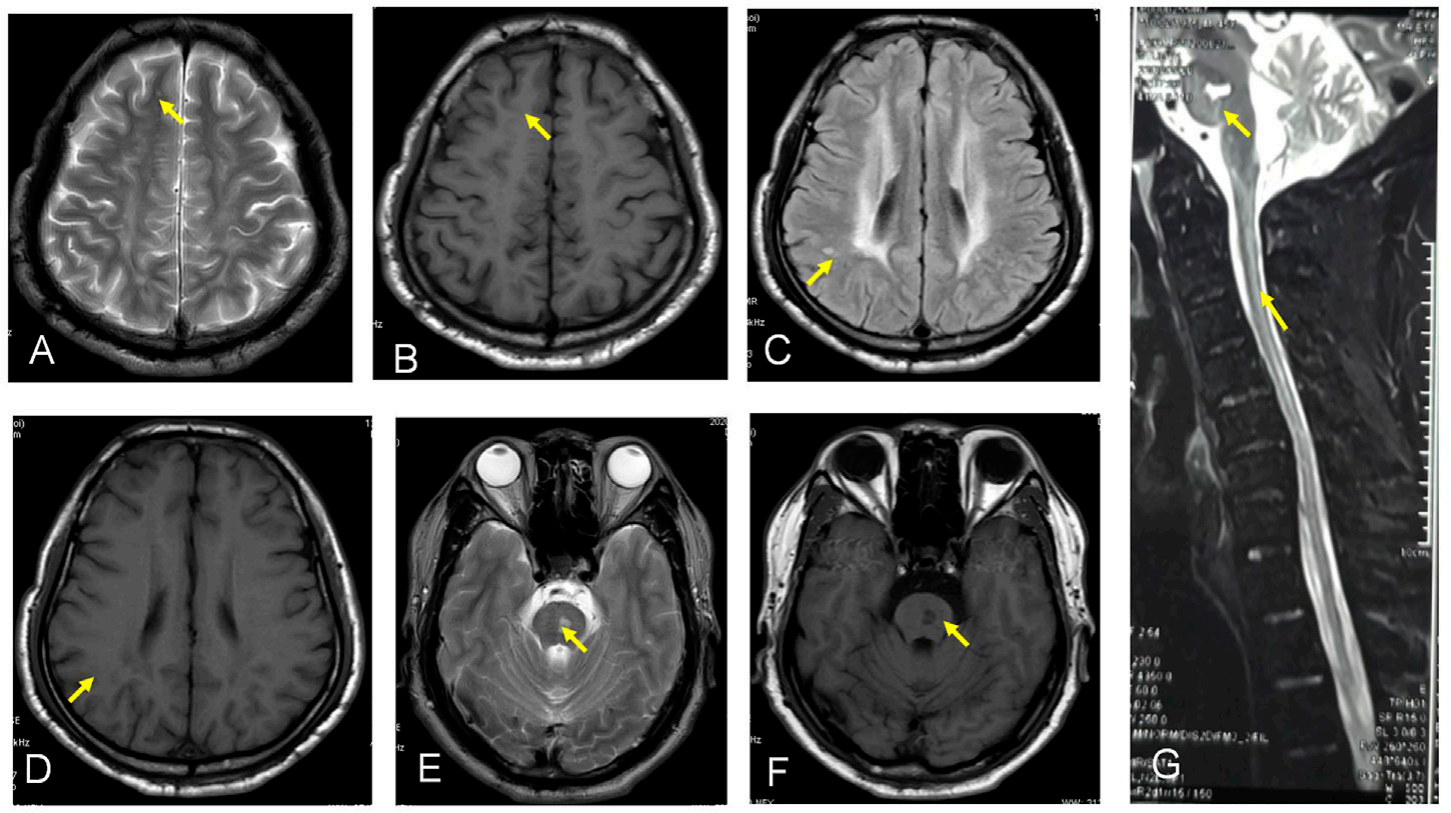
(A–G) show the MRI by a decreased signal on the T1 sequence and increased signal intensity on the T2/FLAIR sequance showed a of the bilateral basal, certain ovale, frontal lobe, and parietal lobe, and F shows increased signal on the T2 sequence showed a of ganglia cervical cord.
